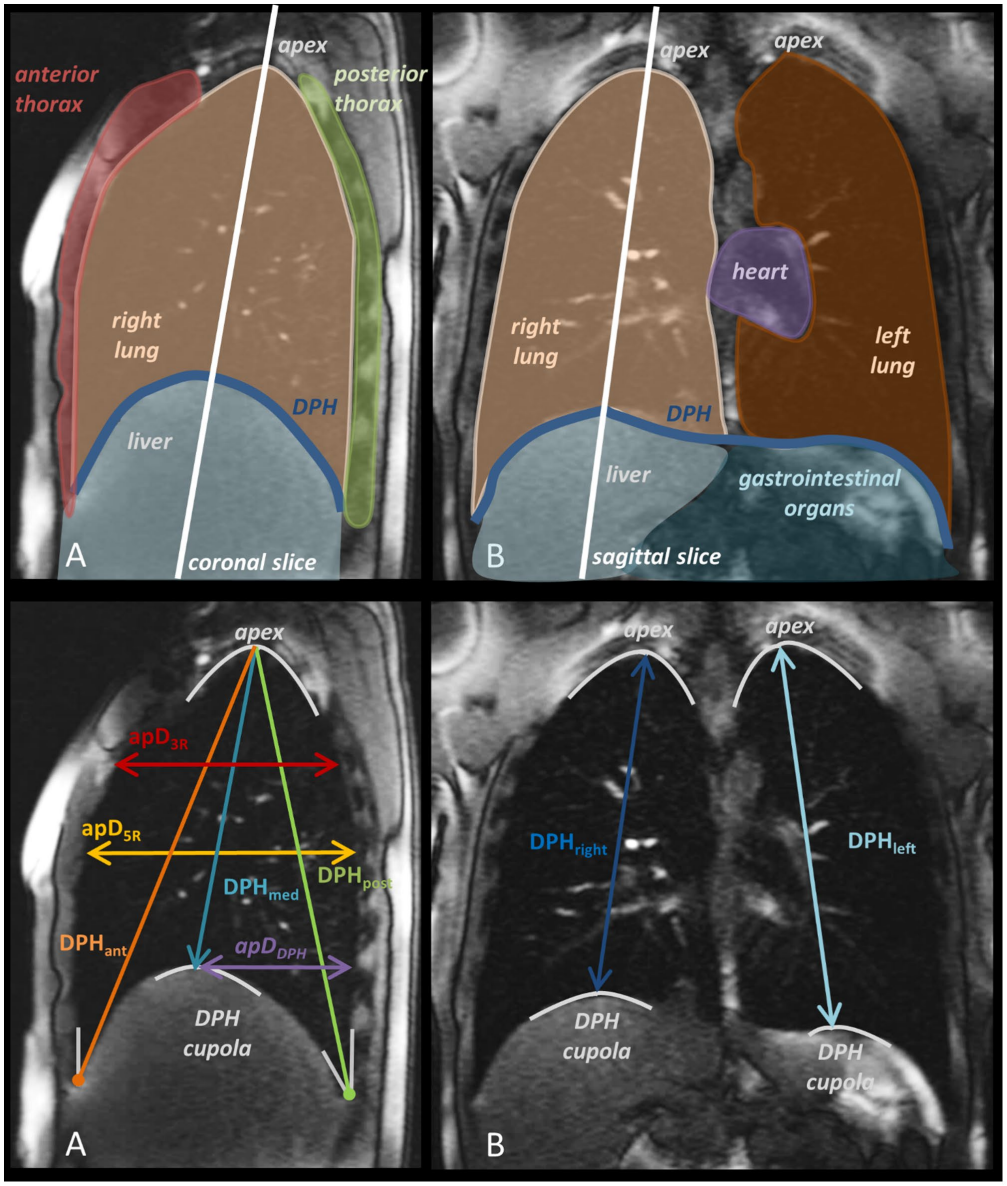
Sagittal (A), and coronal (B), MR image with anatomical designations and slice orientation (upper row) and measured distances with their definition according to anatomical landmarks (lower rows).
